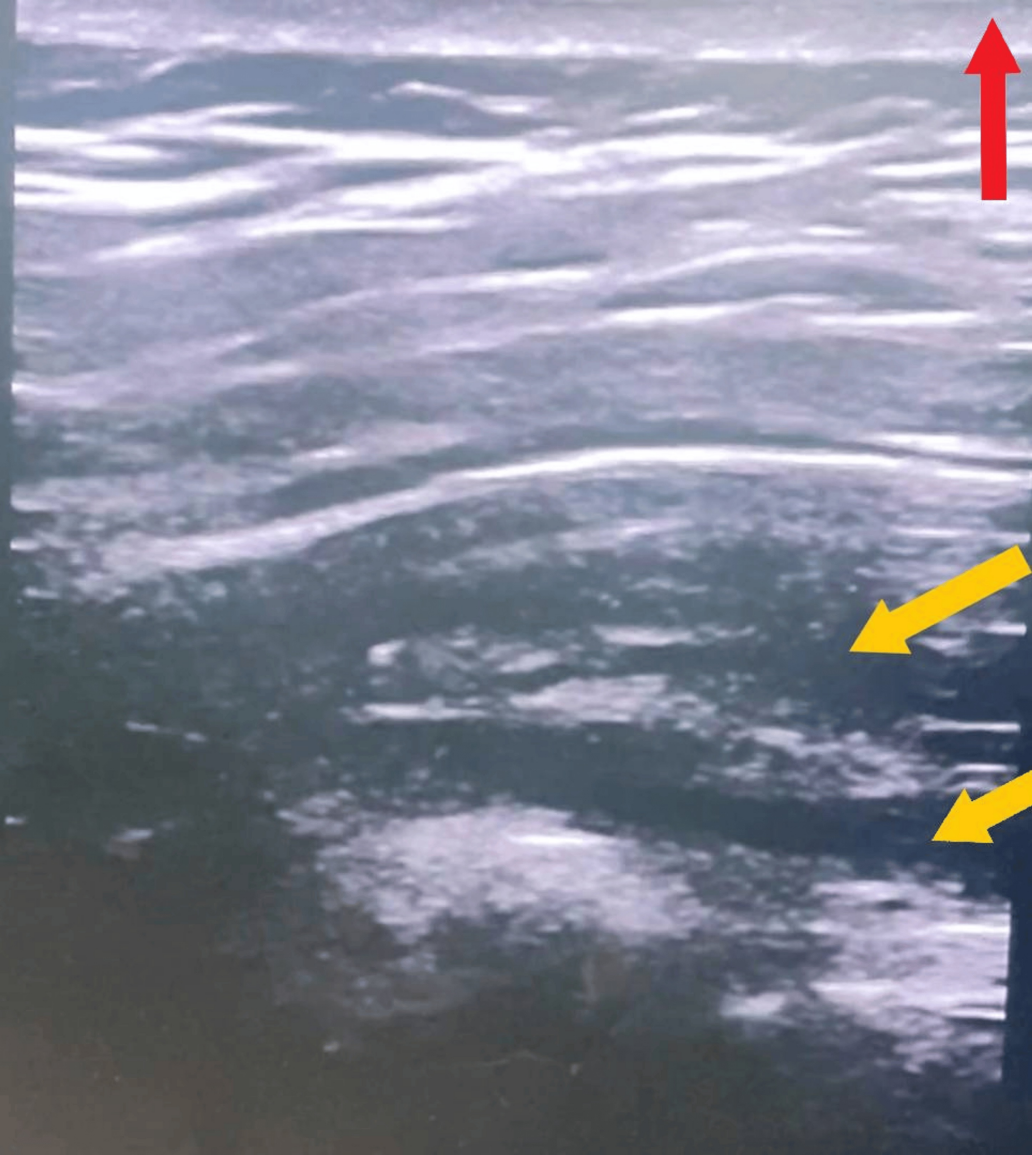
Ultrasound view of the paravertebral block being administered Yellow arrows show the local aesthetic injected expanding between tissue planes. The red arrow points to the skin surface.
